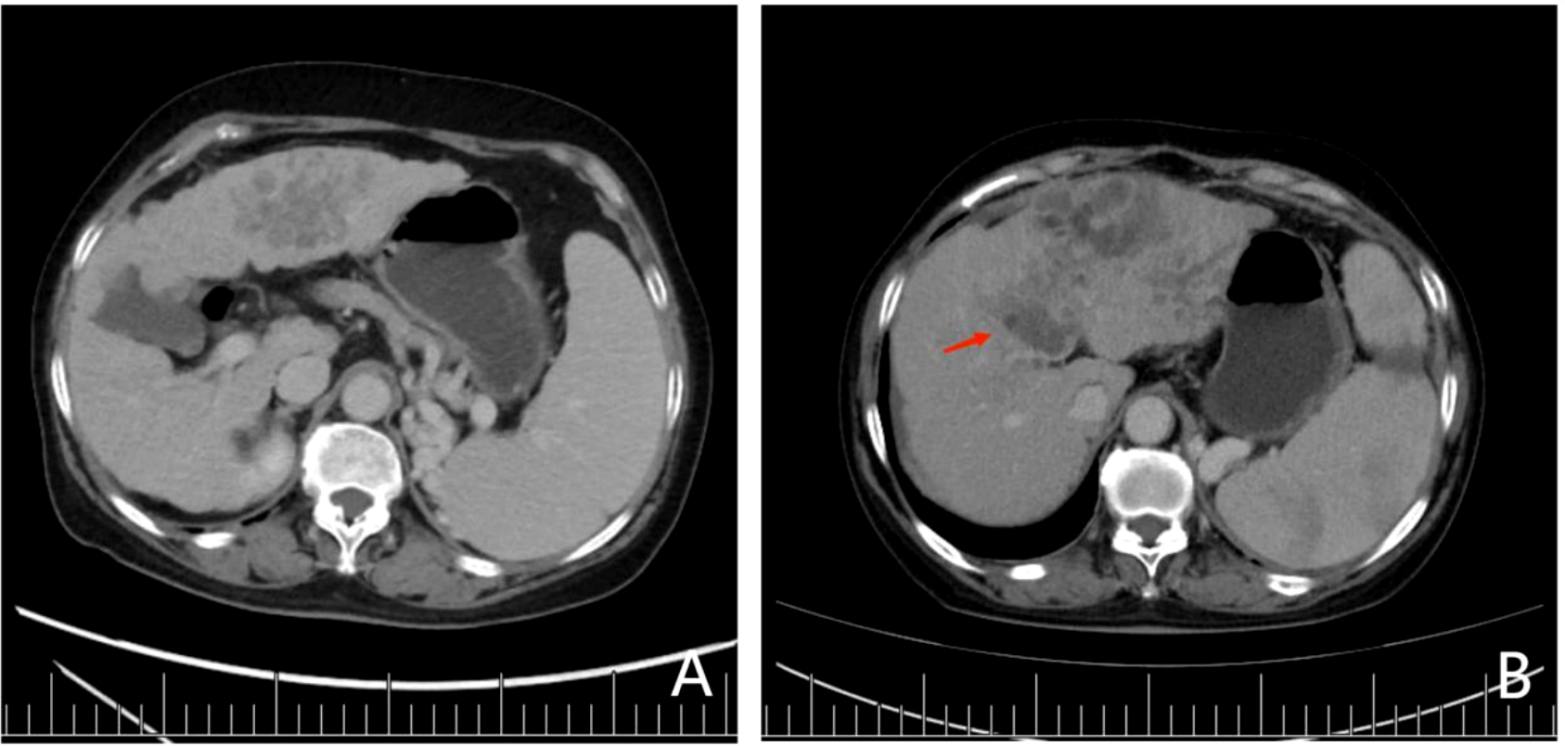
(A) Preoperative contrast-enhanced CT showed two lesions in the patient’s liver with cirrhosis. The largest lesion was 7.42 cm in diameter, located in the left lobe of the liver. In contrast-enhanced CT, lesions in the arterial phase were significantly enhanced, although they were weakened in the delayed phase, which was in accordance with the diagnostic criteria for hepatocellular carcinoma. Portal vein blood flow was unobstructed, and no portal vein tumor thrombus was found. (B) After 2 cycles of TACE treatment, lesions in the left hepatic lobe were enlarged, and tumor thrombus was seen in the portal vein (red arrow).
